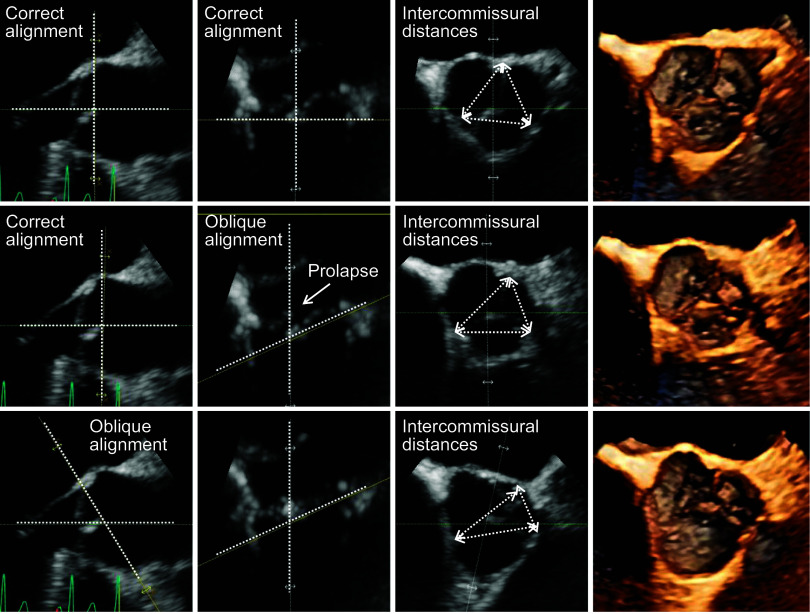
The use of two (2-D)- and three (3-D)-dimensional echocardiography to perform aortic valve apparatus (AVA) measurements, such as the intercommissural distances. Adapted from Ref. 232, with permission per Open Access terms.
